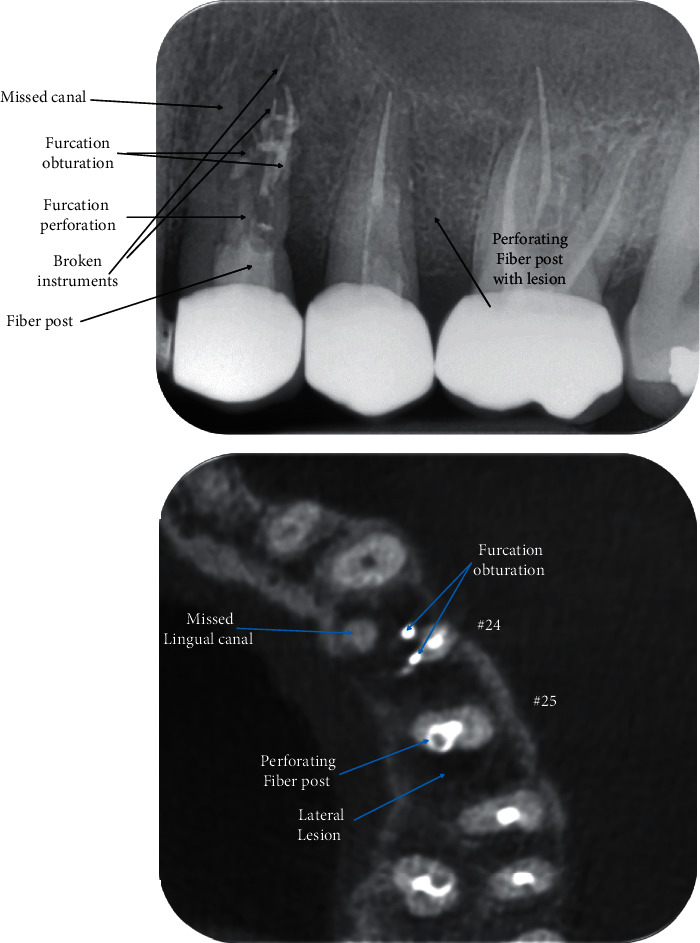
The initial periapical radiography and the axial section of CBCT scans of the teeth #24 and #25.
